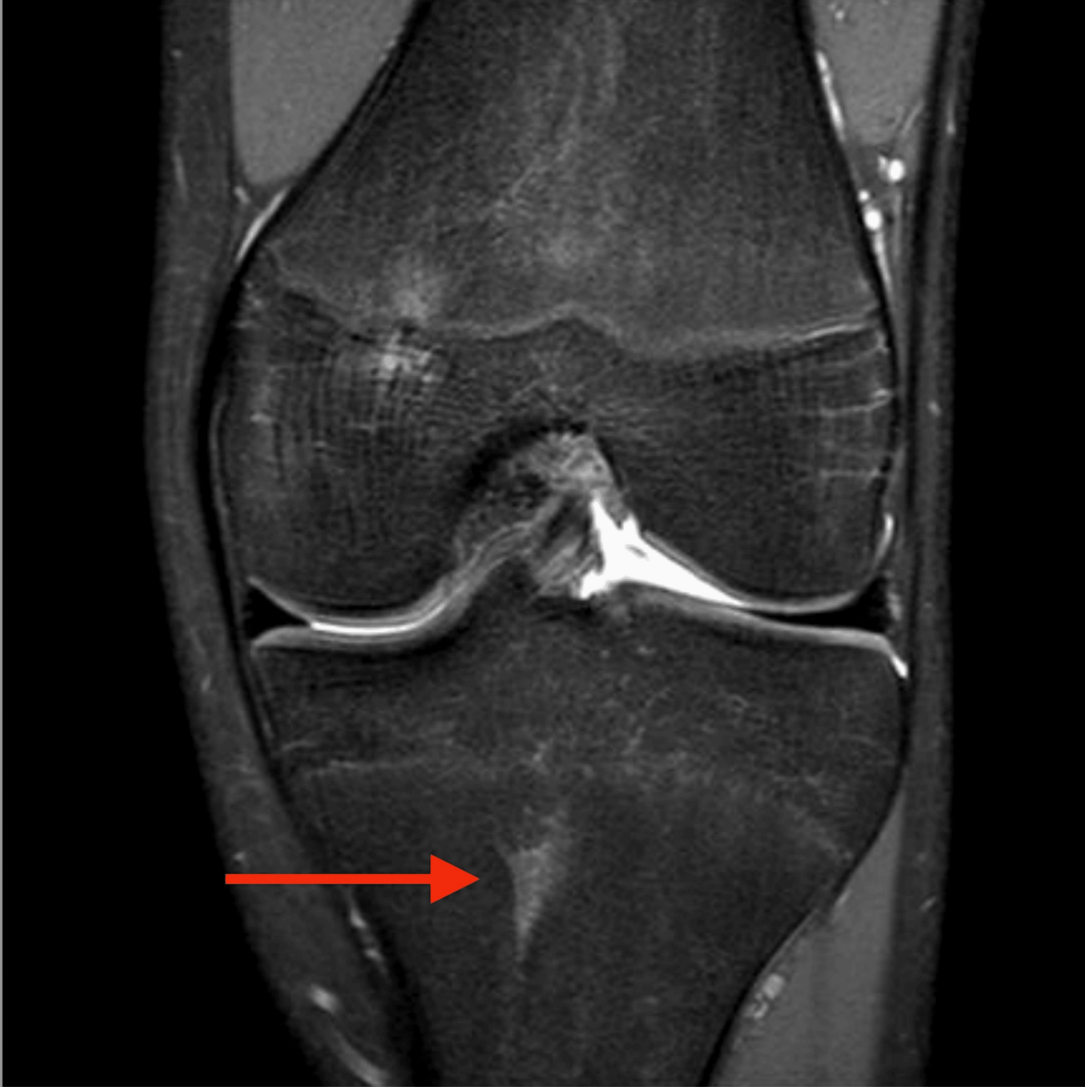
T2-weighted, fat-suppressed anteroposterior view of the knee showing hyperintense signals in the proximal tibial physis (arrow).
